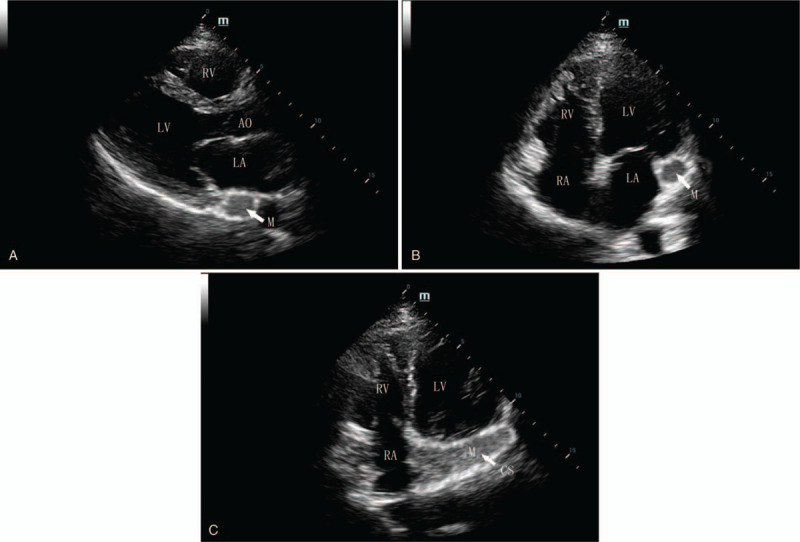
(A) TTE showing a dilated coronary sinus (CS) filled with a hypoechoic mass in the parasternal long axis view. (B) The CS tumor was detected in the apical four-chamber view. (C) A low echo mass extended from the CS to the right atrium in the non-standard four-chamber view. AO = aorta, CS = coronary sinus, LA = left atrium, LV = left ventricle, M = mass, RA = right atrium, RV = right ventricle, TTE = transthoracic echocardiography.
